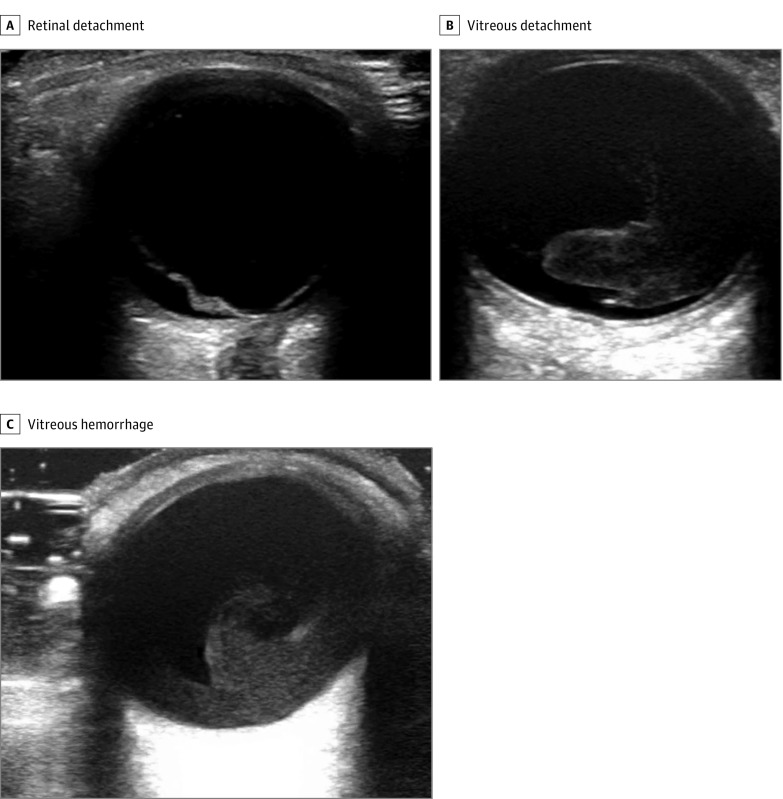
Point-of-Care Ultrasonographic Images A, Retinal detachment. A bright, echogenic membrane is tethered to the optic disc but separated from the choroid in the far field of the image. B, Vitreous detachment. A detached, thin, mobile membrane can be seen at the interface between the vitreous and the retina. C, Vitreous hemorrhage. A fluid collection of variable echogenicity can be seen in the posterior chamber.
